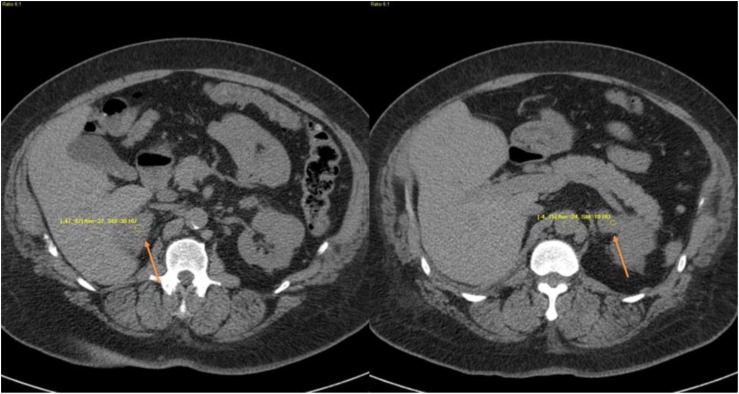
CT images from case 2 showing diffuse bilateral enlargements (arrows).
